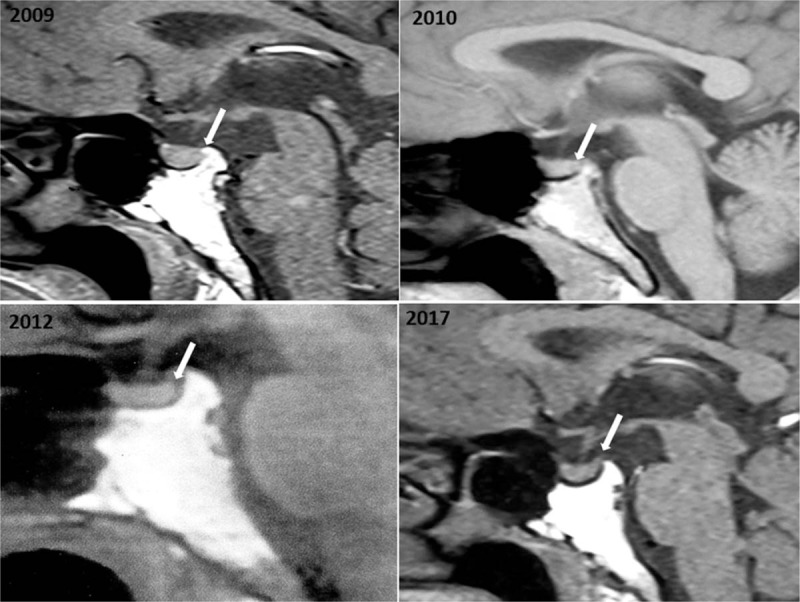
Follow-up magnetic resonance image of pituitary gland in several years. The bright spot was always loss in the posterior of pituitary gland.
